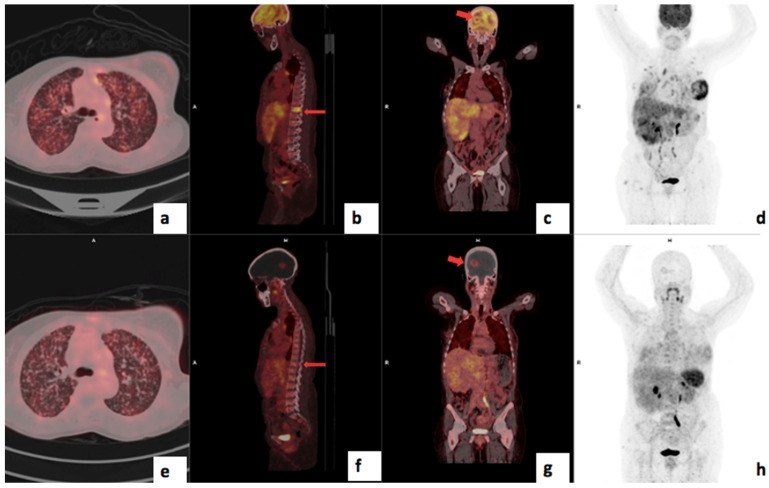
A 48-year-old female with left T3N1M1 breast cancer showing increased heterogeneous uptake in the left breast primary (SUVmax 13.1), with brain, liver, lung and skeletal metastasis using ^18^F-FDG PET/CT (a–d). The lesions are seen on ^68^Ga-Pentixafor (e–h) but appear less intense (SUVmax 4.26). The brain lesion is more visible using Pentixafor (g) due to lower brain uptake than FDG (c) and the vertebral lesion is more visible on FDG (b) than Pentixafor (f) due to higher physiological marrow uptake (h).
